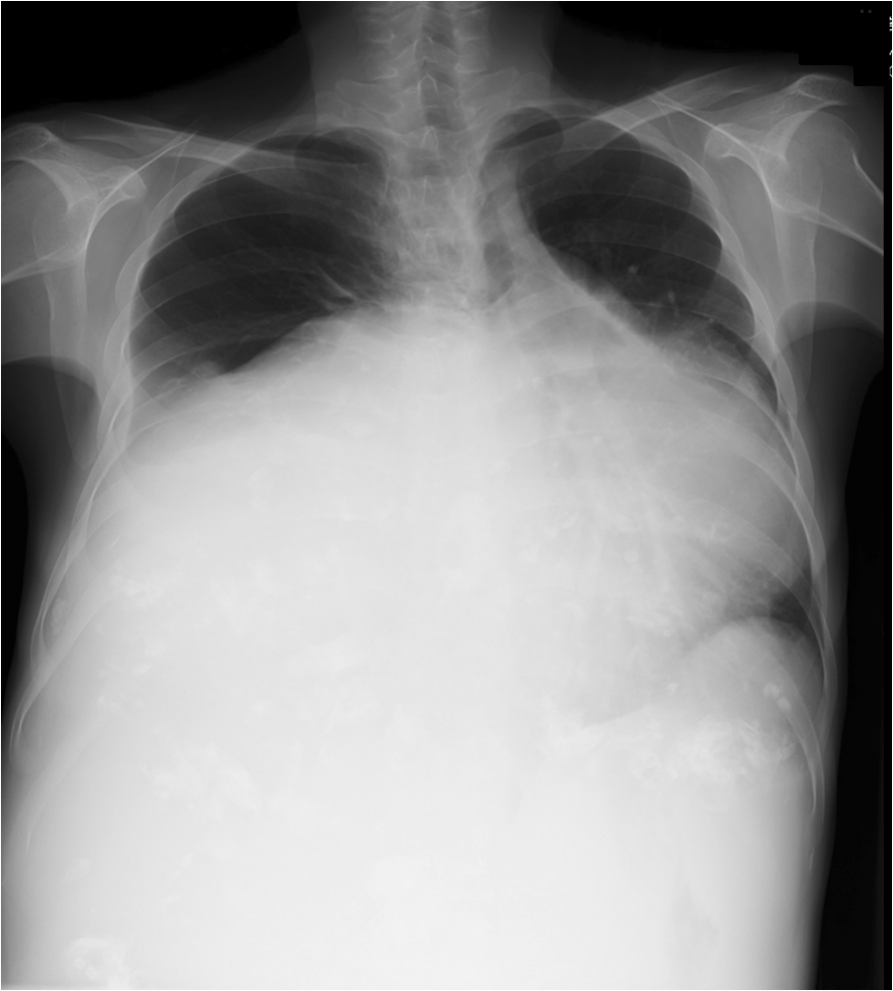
Preoperative chest X-ray showing bilateral diaphragmatic elevation because of the tumor.
